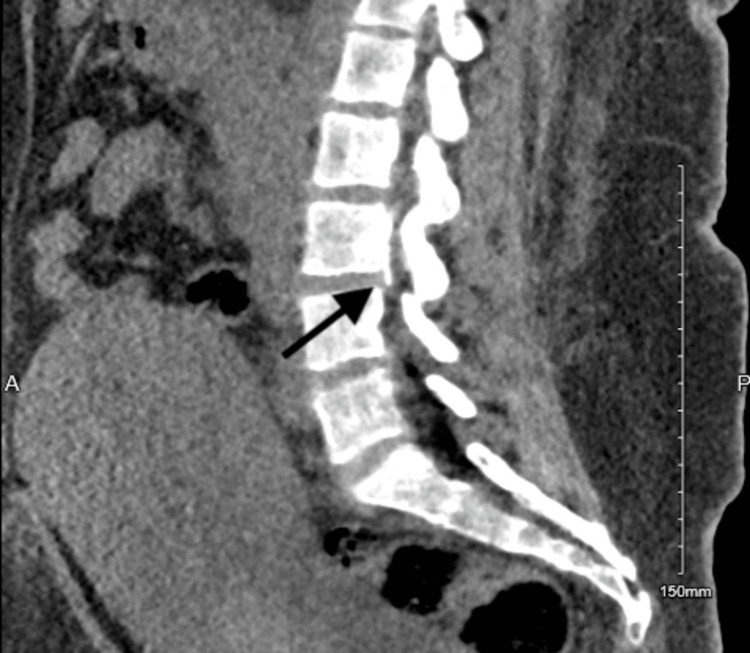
CT scan of the spine of the lumbar (at the L2 level). The CT scan sequence indicates the epidural catheter's entry and where it ends. Here you can note that one is superficial at the level of the L2 vertebra, measuring 1.2 cm, and the other is deep into the paraspinous muscle with extension to the spinal canal at the L2-L3 level.
